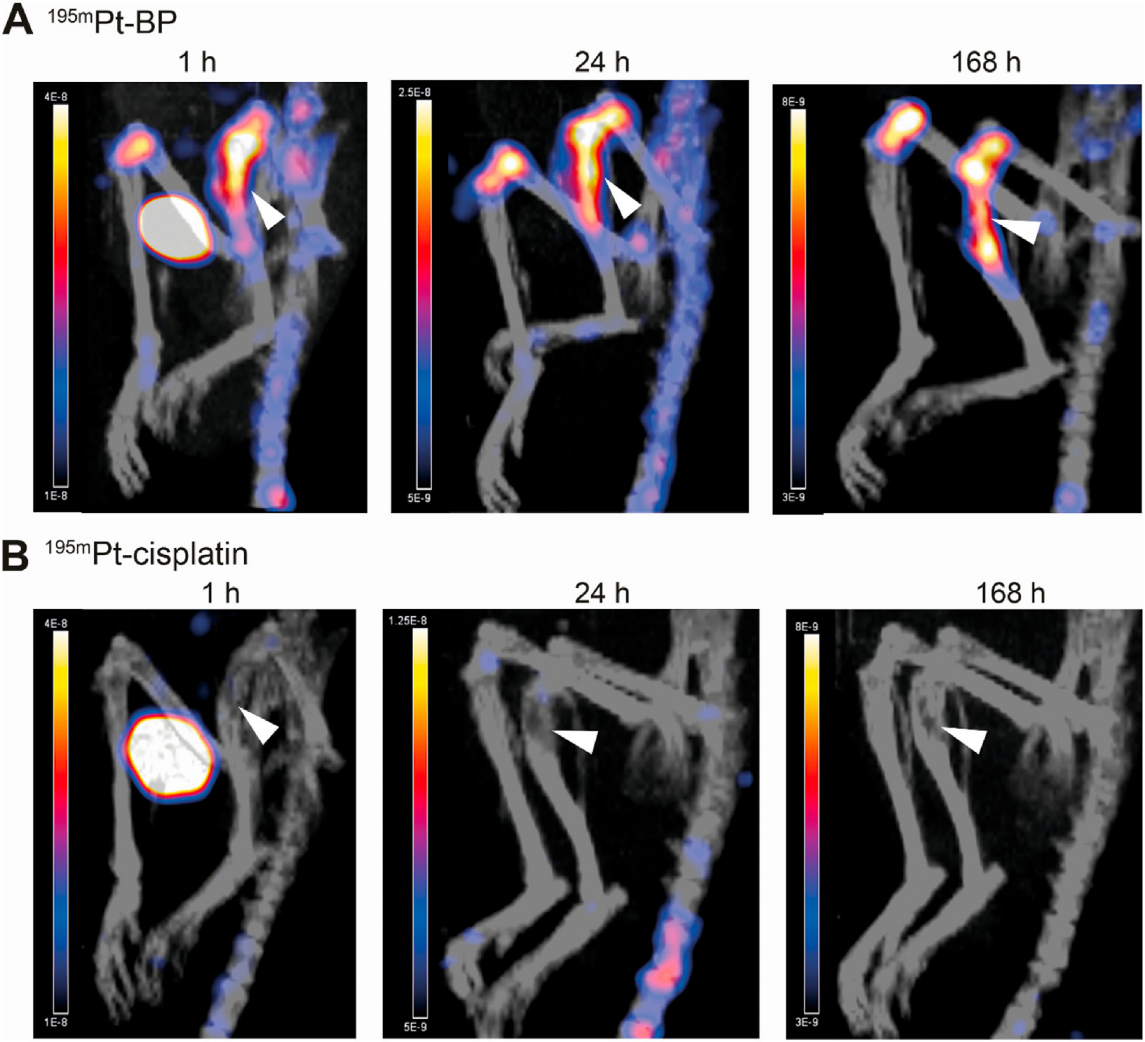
Biodistribution of ^195m^Pt-BP and ^195m^Pt-cisplatin in prostate cancer cell–induced intratibial lesions. Representative micro-SPECT/CT images showing biodistribution of ^195m^Pt-BP (A) and ^195m^Pt-cisplatin (B) in control tibia (left) and tibial lesion (right) at 1 ​h, 24 ​h, and 168 ​h after systemic administration. White arrowheads correspond to the tibial lesions.
